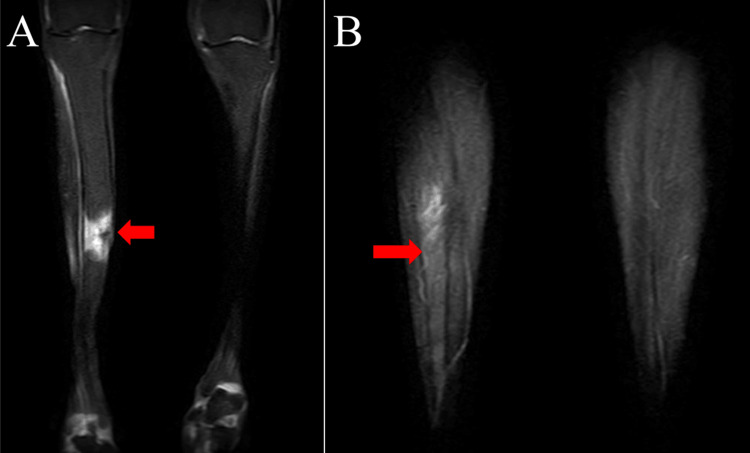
Preoperative short-tau inversion-recovery (STIR)-MRI findings (A) Coronal STIR image showing a high STIR area in the anterior tibial cortex and medullary cavity (red arrowhead), consistent with marrow edema and inflammation; (B) Axial STIR image demonstrating cortical irregularity with matching medullary hyperintensity precisely at the historical snakebite site (red arrowhead).
